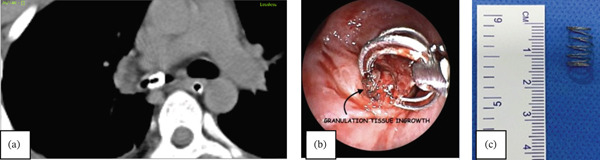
(a) Axial chest CT image showing a metal‐appearing FB in right intermedius bronchus. (b) Metallic foreign body embedded in granulation tissue (c) Retrieved metallic spring FB.
